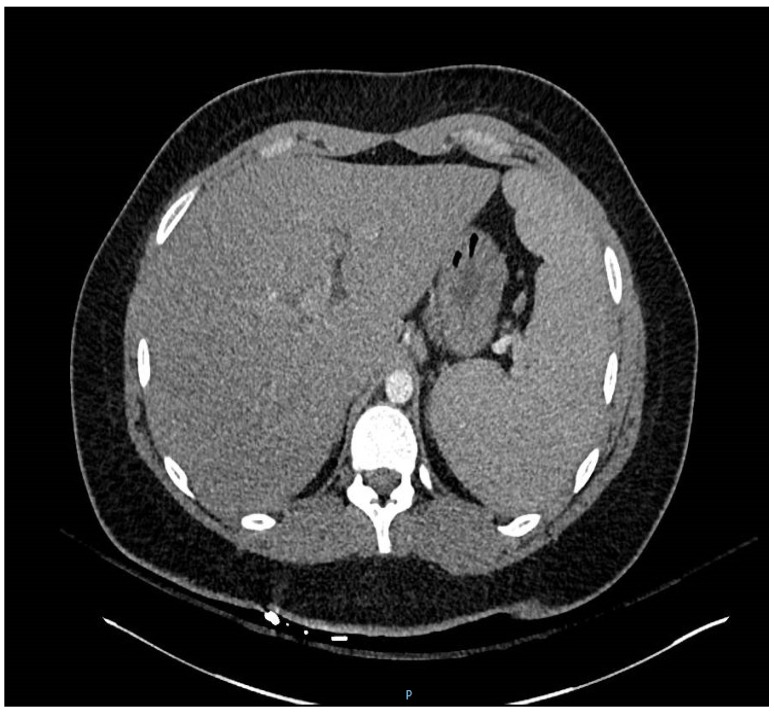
Chest computed tomography (CT) scan showing marked splenomegaly.
